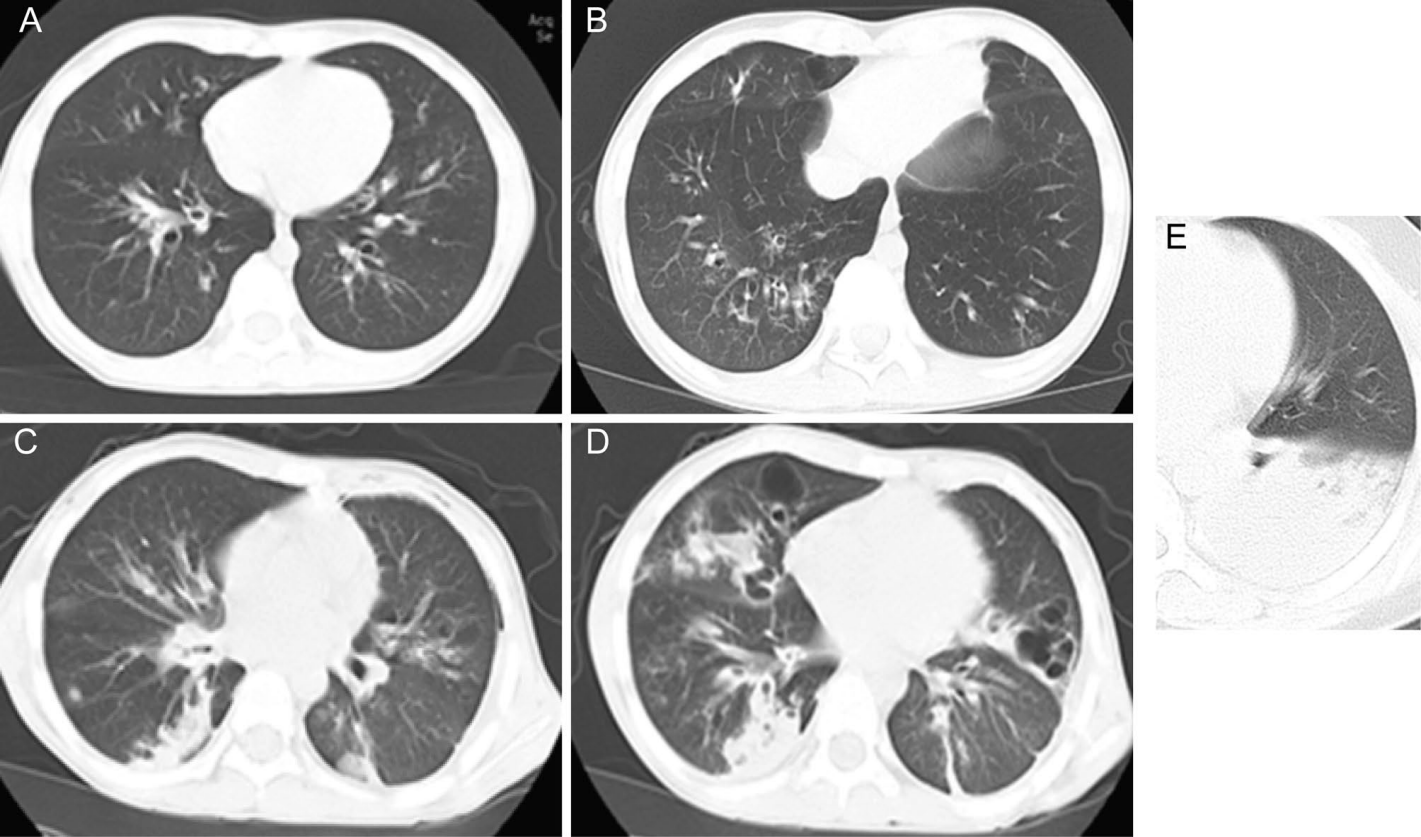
Typical CT images of the CT score. A: CT score was 1; B: GGO involvement was less than 25%, and CT score was 2 points; C: Right inferior lobe of lung: <50%, consolidation, CT score 4 points; Left inferior lobe: GGO, 26–49%, 3 points.; D: Right inferior lobe of lung, 50–75% area involved, consolidation, CT score 6 points; E: Left inferior lobe consolidation > 75%, CT score 7 points
